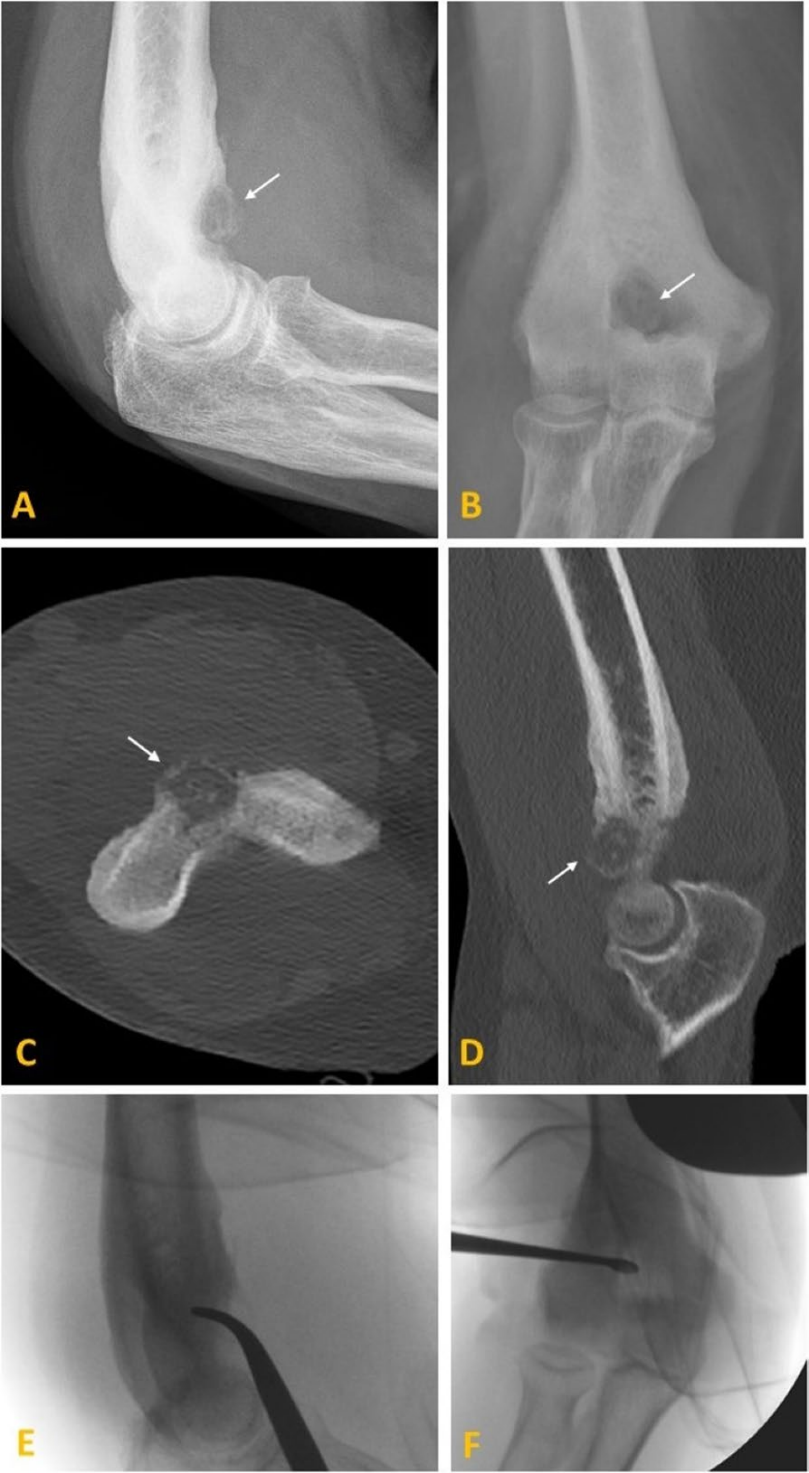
Curettage of an osteoid osteoma of the distal humerus in a 53-year-old female patient. Preoperative radiographs in lateral (A) and AP (B) projections show a juxta-articular geographic osteolytic lesion in the coronoid fossa (arrow) with surrounding reactive sclerosis and circumferential solid periosteal reaction. Corresponding high-resolution CT images in the bone window in axial (C) and sagittal (D) planes additionally show a thin neocortex formation and intralesional calcification. Intraoperative radiographs in lateral (E) and AP (F) projection during curettage
